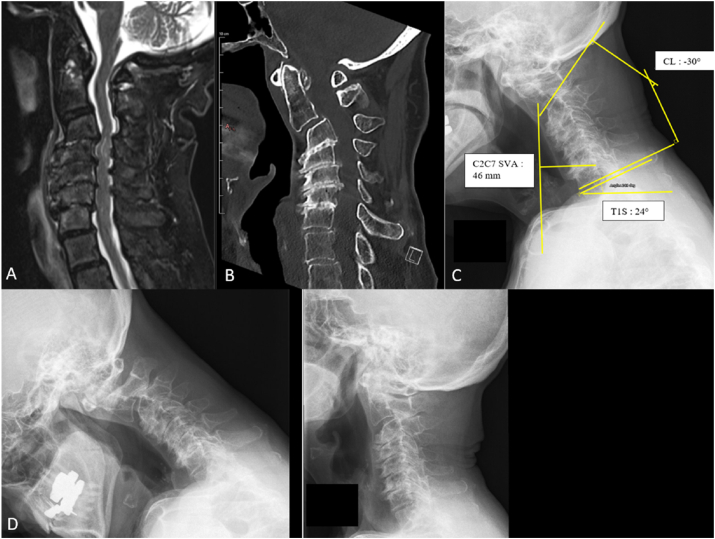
Pre operative MRI (T2 Sagittal- A) CT scan (B) and Standing Radiographs (C) showing preoperative sagittal alignment parameters. Here, the SVA is estimated at 46 mm, indicative of cervical malalignment in the context of extreme cervical kyphosis (−30° for the CL angle) with a T1S – CL mismatch of 54°. Dynamic radiographs (D) reveals the microinstability of the segments C2 to C4.
